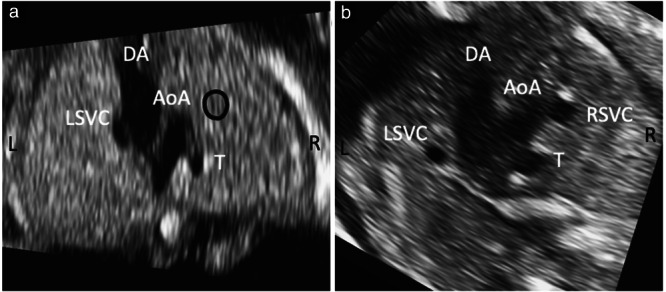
Ultrasound cross‐sectional images at the level of the three‐vessel‐and‐trachea view in a case with single left superior vena cava (LSVC) (a) and a case with bilateral superior vena cava (b). Note that in (a), the fluid‐filled space to the right of the aorta corresponds to the trachea (T), which should not be mistaken for the right superior vena cava (RSVC). The trachea shows an echogenic ring and occupies a more posterior position than that expected for the RSVC, the absence of which is indicated by the black circle. AoA, aortic arch; DA, ductus arteriosus; L, left; R, right.
